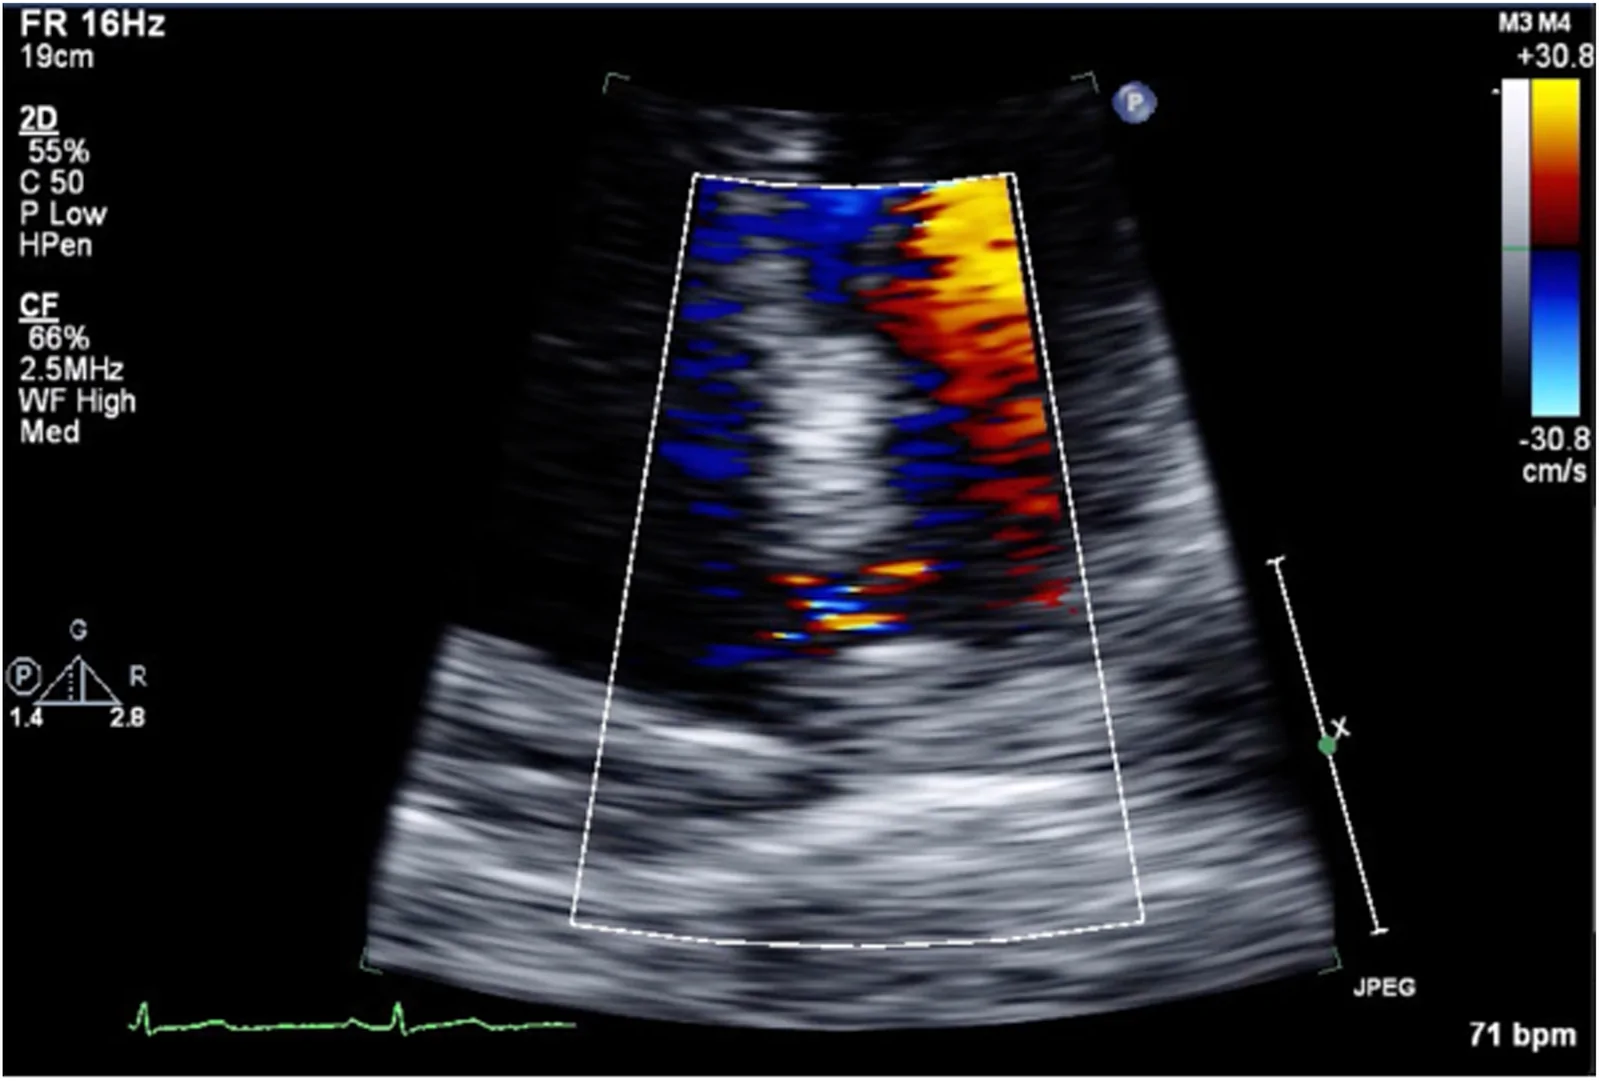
Transthoracic echocardiogram demonstrating patent foramen ovale with transient right-to-left shunt on zoomed-in apical 4-chamber view.
